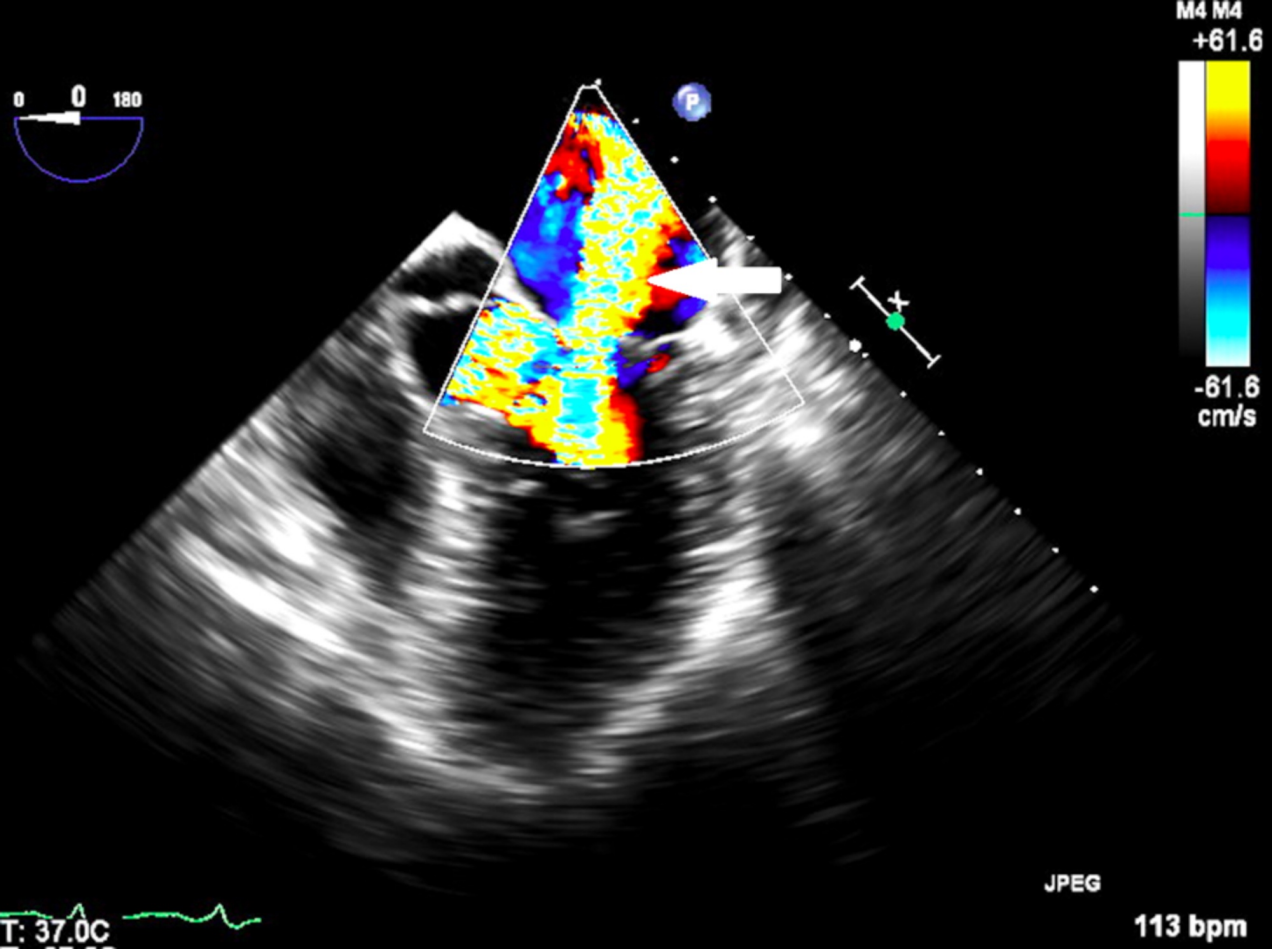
Transesophageal echocardiographic image showing mitral regurgitation with color Doppler (white arrow)
